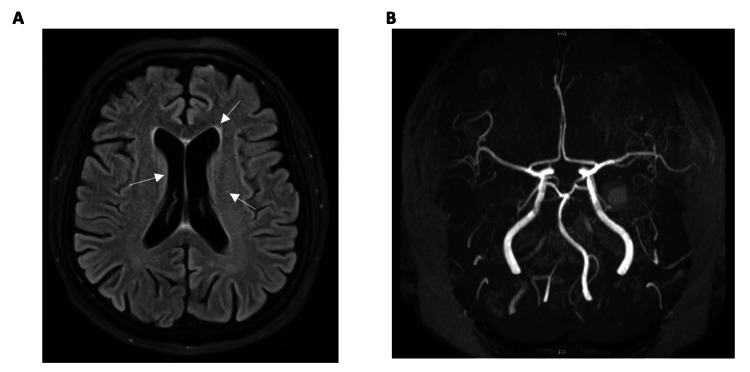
Magnetic resonance imaging (MRI) of the brain showed a few discrete hyperintensities in the bilateral periventricular region (A), with unremarkable findings on the magnetic resonance angiogram (MRA) (B)
